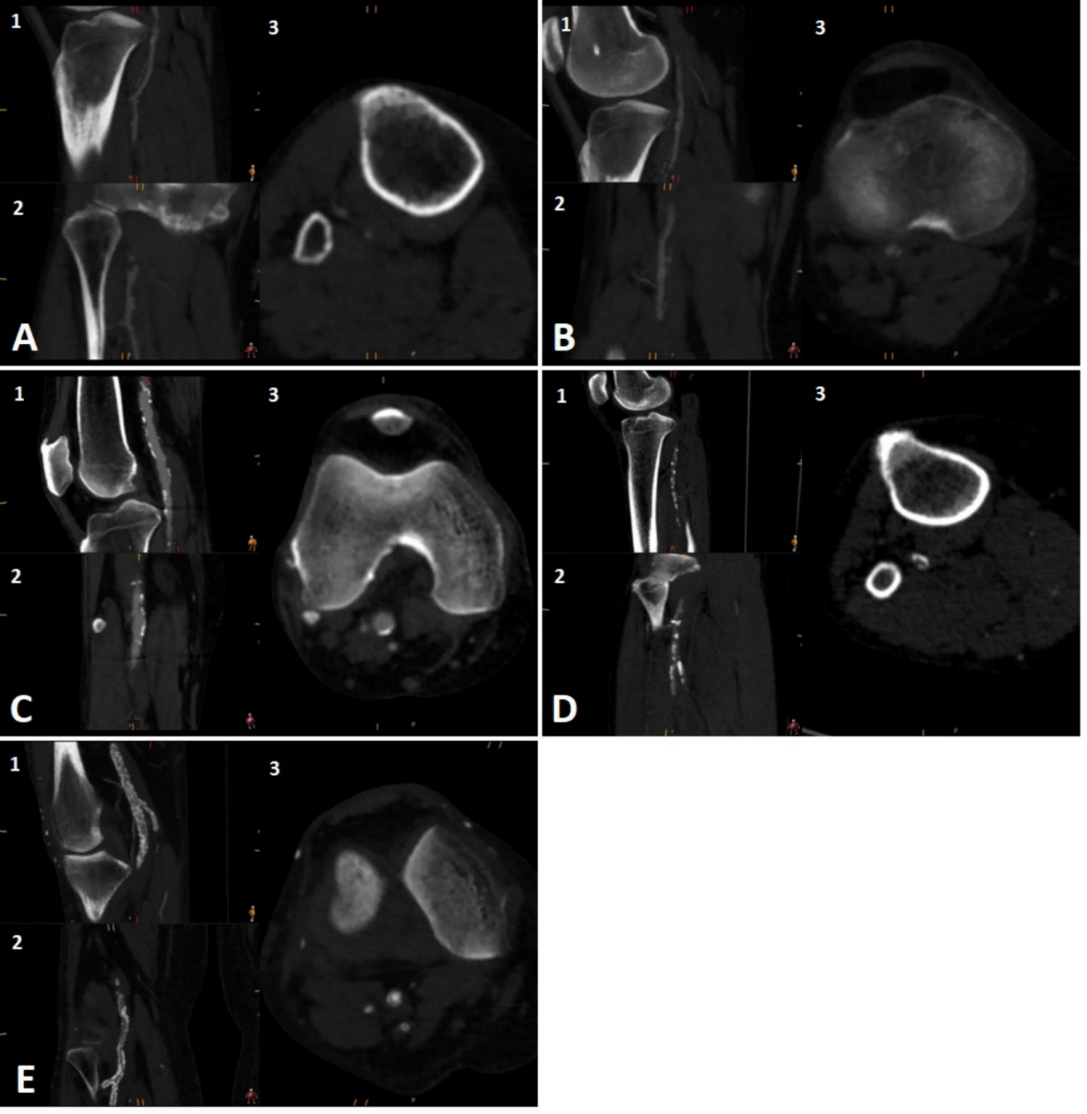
Computed tomography angiography (CTA) examples of the different peripheral arterial calcification scoring system (PACSS) grades. The figure shows sagittal (1), coronal (2) and transversal (3) planes of limbs with PACSS 0 (A), PACSS 1 (B), PACSS 2 (C), PACSS 3 (D) and PACSS 4 (E)
